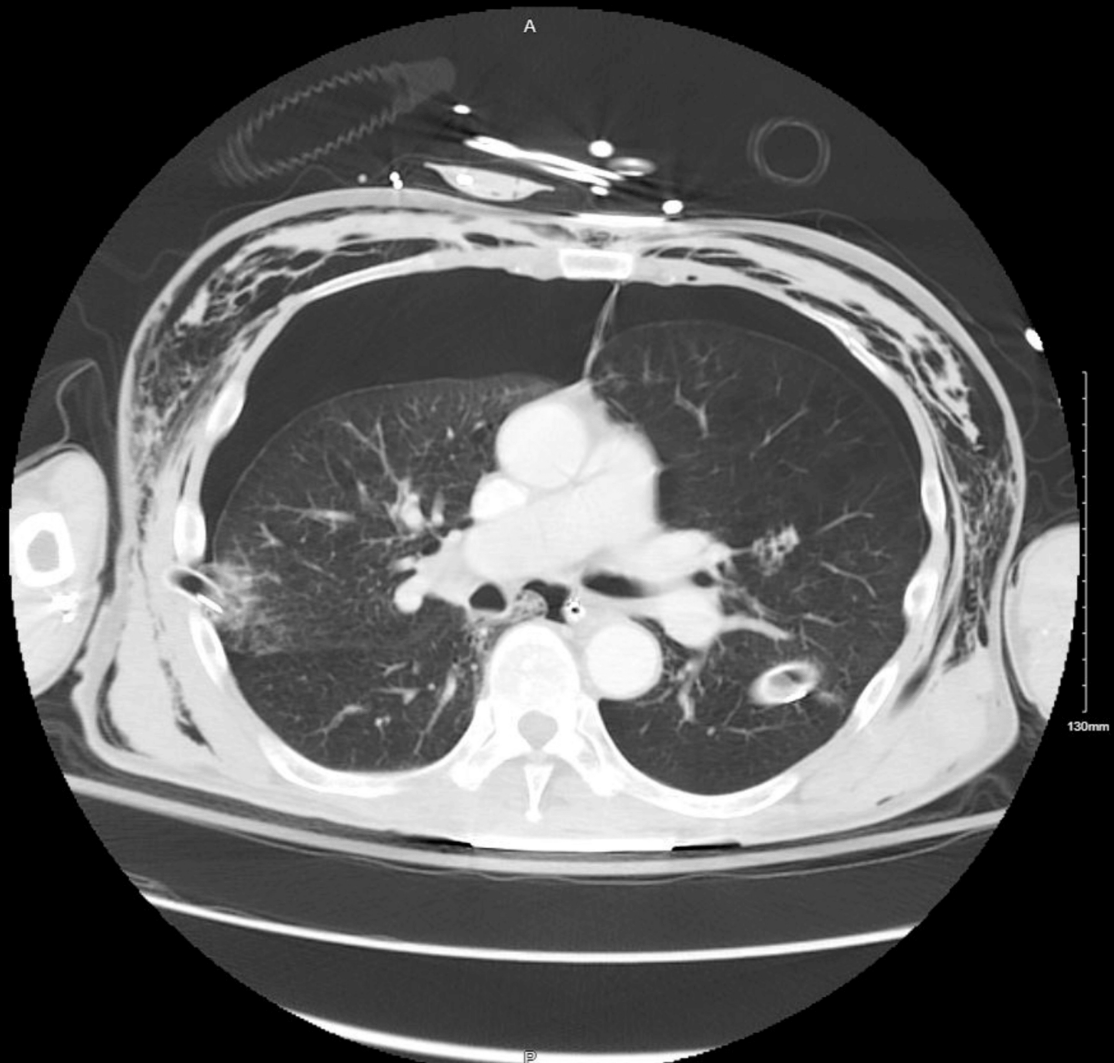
An initial CT scan of the chest demonstrates bilateral pneumothoraces. Of note, the left chest drain is potentially penetrating the left lung parenchyma.
